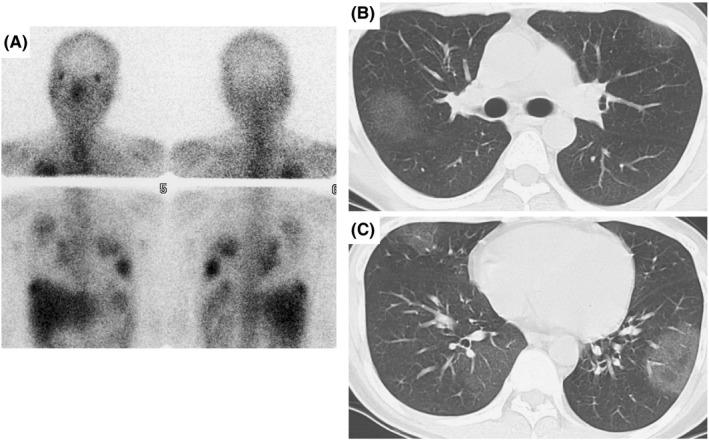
Imaging findings. A, Gallium‐67 scintigraphy showed bilateral segmental lung uptake, and no uptake in kidney; B and C, Chest computed tomography revealed bilateral segmental ground grass opacities
